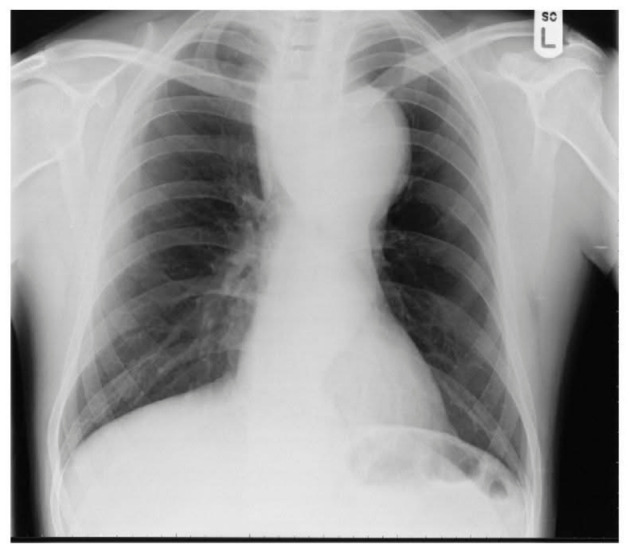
Anteroposterior chest radiograph demonstrating dilation of the aortic arch with contralateral tracheal displacement. Although chest X-ray cannot replace CTA for detailed assessment, it may provide an initial clue to underlying aortic pathology in patients presenting with acute chest pain, prompting further cross-sectional imaging.
